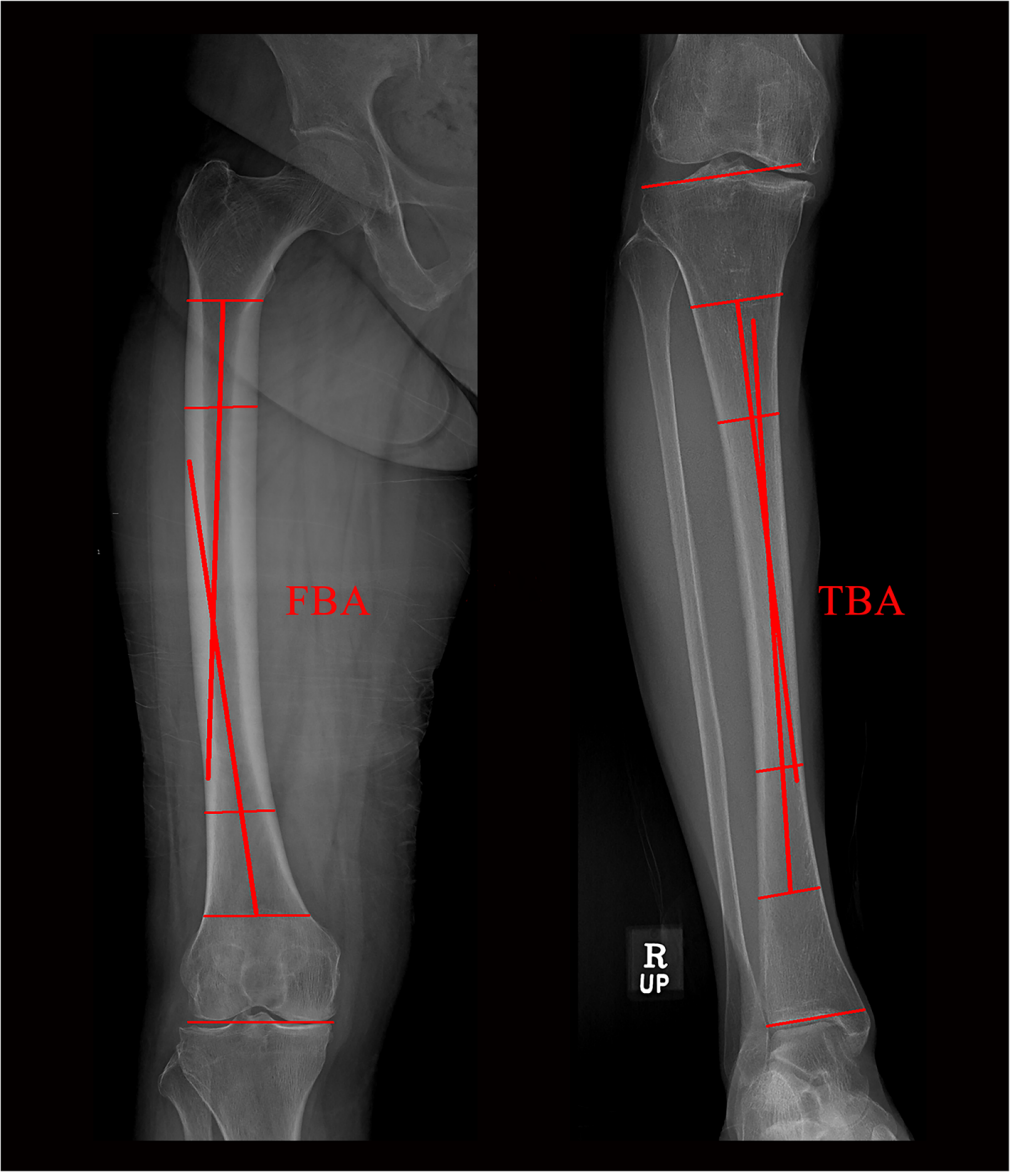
Preoperative full-length (14 × 51 in. grid cassette) radiograph showing the measurement of the femoral bowing angle (FBA) and tibial bowing angle (TBA). The FBA was defined as the angle between the line connecting the points bisecting the femur at 0 cm and 5 cm below the lowest portion of the lesser trochanter and the line connecting the points bisecting the femur at 5 cm and 10 cm above the lowest portion of the lateral femoral condyle [23]. The TBA was defined as the angle between the line connecting the points bisecting the tibia at 5 cm and 10 cm from the highest portion of the lateral tibial plateau and the line connecting the points bisecting the tibia at 5 cm and 10 cm from the lowest portion of the tibial plafond [19].
